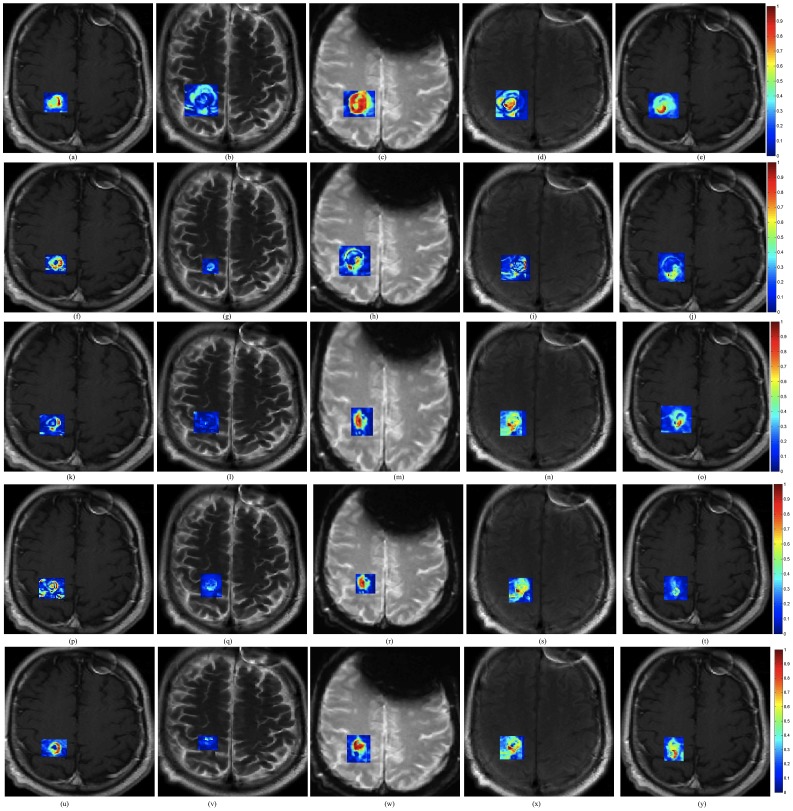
(a)–(e) Difference maps between 24-hours post-LITT and pre-LITT (baseline) for T1w (a), T2w (b), FLAIR (c), and GRE (d). Figs. 10(f)–(i) show difference maps for 2-month post-LITT with respect to baseline, (k)–(n) show difference maps for 7-month post-LITT, (p)–(s) show difference maps for 9-month post-LITT and (u)–(x) show difference maps for 11-month post-LITT with respect to baseline for T1w, T2w, GRE and FLAIR respectively. Corresponding fused multi-parametric MRI maps obtained by weighted combination of individual MRI protocols are shown in Figs. 10(j), (o), (t), (y), corresponding to differences at 24-hour, 2-months, 7-months, 9-months, and 11-months with respect to baseline scan respectively.
